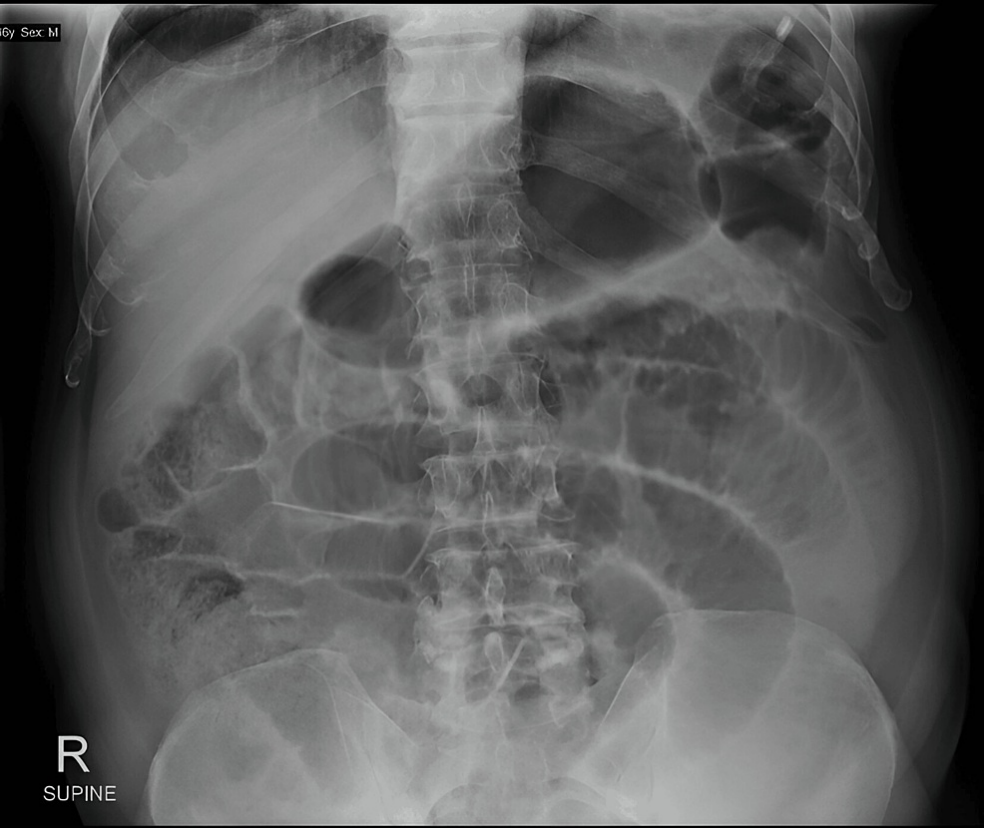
An abdominal X-ray revealed multiple dilated small bowel loops of up to 4.7cm in diameter with no evidence of intraperitoneal free gas
